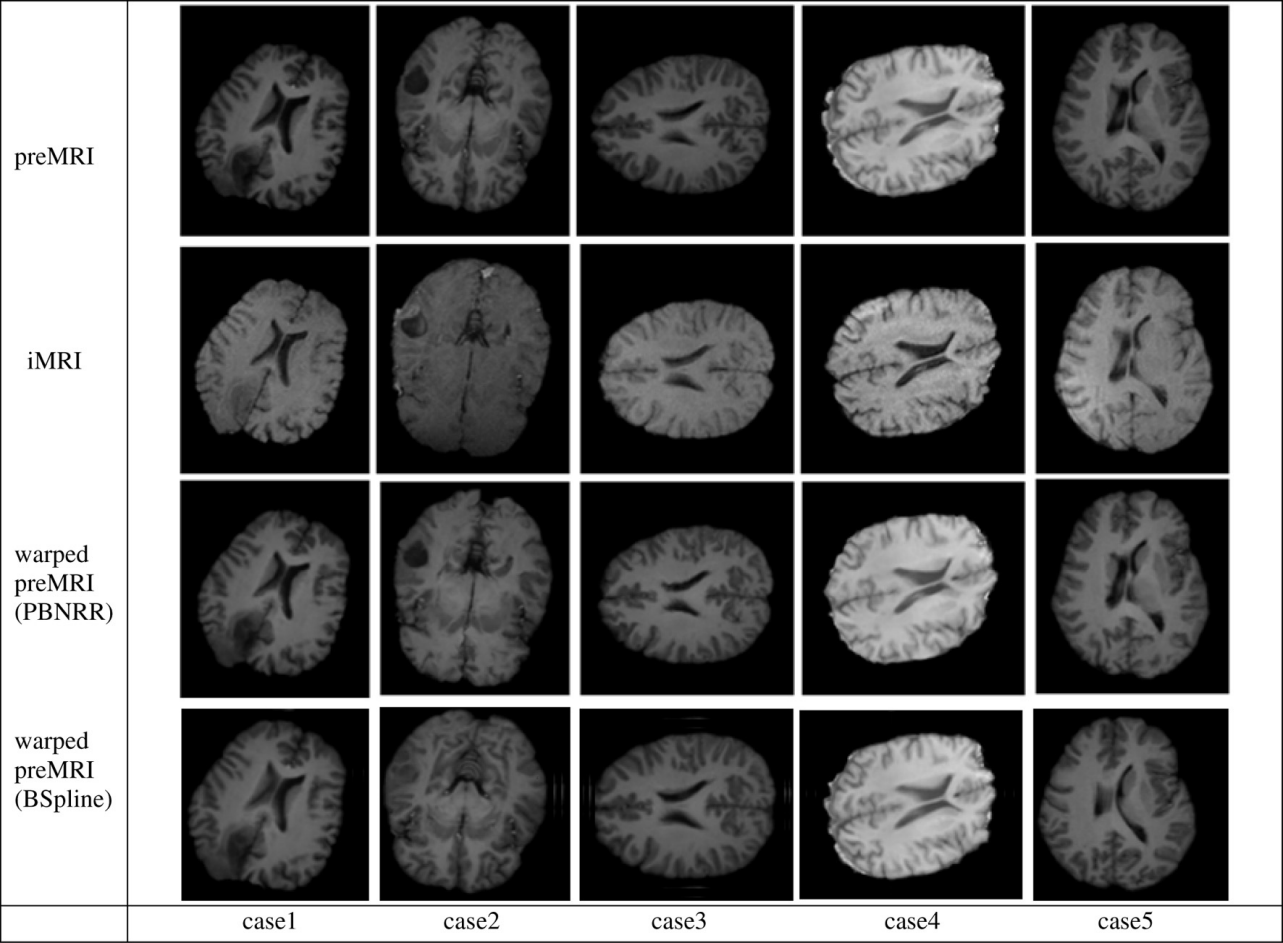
The Qualitative results for the five cases of the PBNRR filter. Each column corresponds to a different case, and each row from the top to the bottom: the preoperative MRI, the intra-operative MRI, and the warped preoperative MRI using PBNRR and the warped preoperative MRI using BSpline based NRR.
